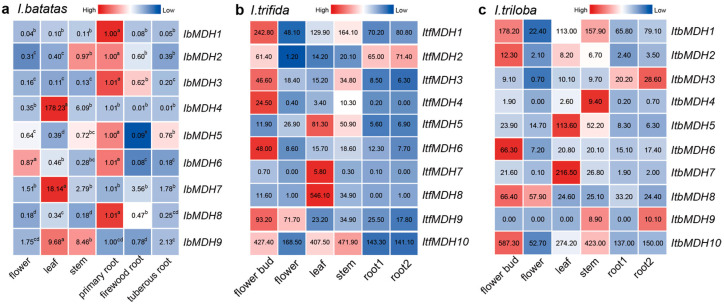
Expression analysis of *MDHs* in different tissues of *I. batatas*, *I. trifida*, and *I. triloba* using RNA-seq. (a) Expression analysis of *IbMDHs* in different tissues. The values were determined via qRT-PCR from three biological replicates consisting of pools of three plants, and the results were analyzed using the comparative CT method. The expression level of each *IbMDH* gene in primary root is set as control. Different lowercase letters indicate a significant difference in each *IbMDH* at *p* < 0.05 based on one-way ANOVA followed by Tukey’s post hoc test. (b) Expression patterns of *ItfMDHs* in the flower bud, flower, leaf, stem, root 1, and root 2 of *I. trifida*. (c) Expression patterns of *ItbMDHs* in the flower bud, flower, leaf, stem, root 1, and root 2 of *I. triloba*. The fragments per kilobase per million (FPKM) values are shown in the color blocks.
